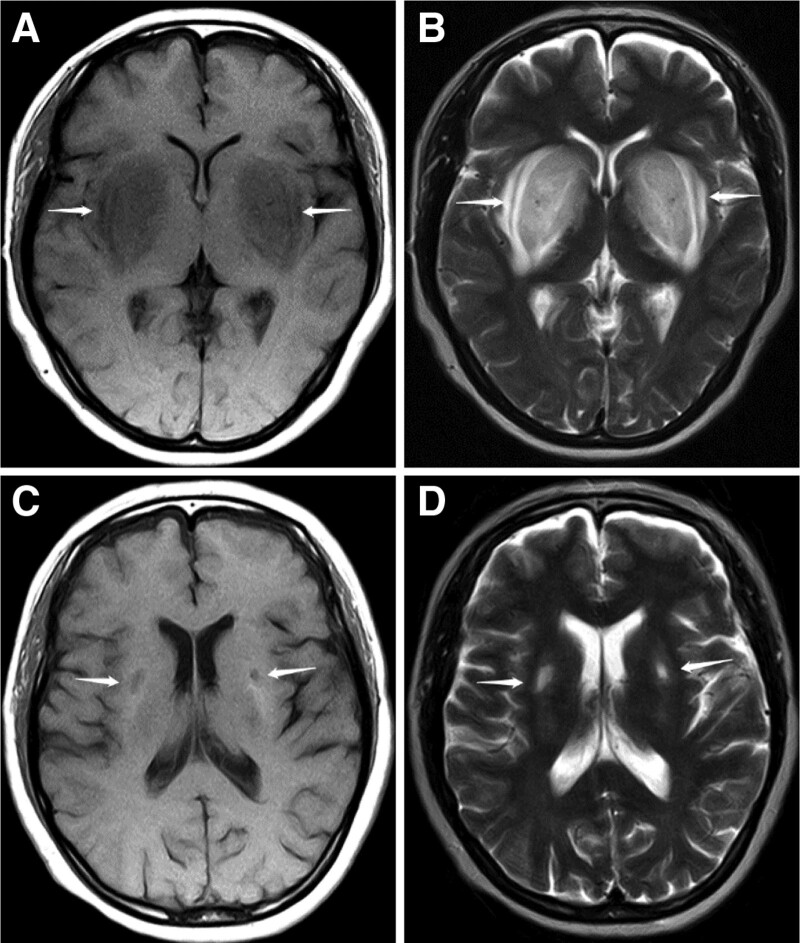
Brain MRI imaging before and after treatment in case 1. (A) T1 image of the brain shows bilateral symmetrical low-intensity signals in basal ganglia (arrow) and (B) symmetrical high signal intensity in bilateral basal ganglia on T2 images of the brain (arrow) before LFS treatment. (C) T1 images of the brain show that bilateral symmetrical signal hypo-intensities were significantly reduced in the basal ganglia with clear margins (arrow), and (D) T2 images of the brain show that the bilateral symmetrical hyperintense signals in the basal ganglia were significantly reduced, with clear margins after receiving treatment. LFS = lentiform fork sign, MRI = magnetic resonance imaging.
